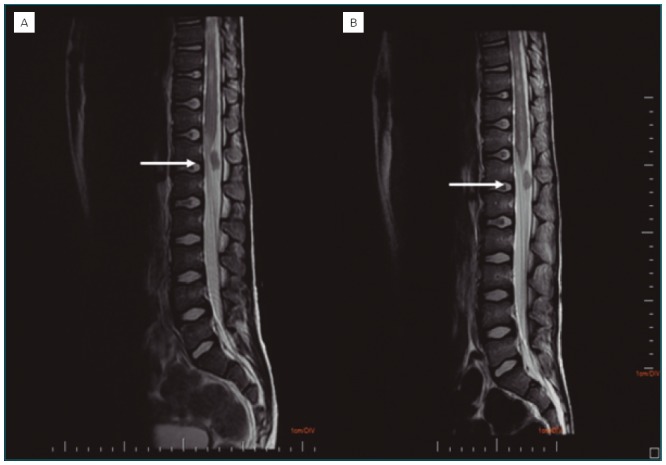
(A) Magnetic resonance imaging of the lumbar spine at the time of diagnosis. The T2-weighted image shows intradural and extramedullary oval formation that promotes displacement of the spinal cord. (B) A control MRI after three months shows no significant changes from the initial exam.
